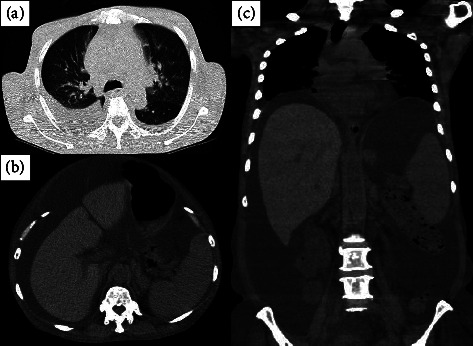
Computed tomography images, (a) lung image in transverse plane, (b) abdominal image in the transverse plane, and (c) thoracoabdominal image in the coronal plane.
